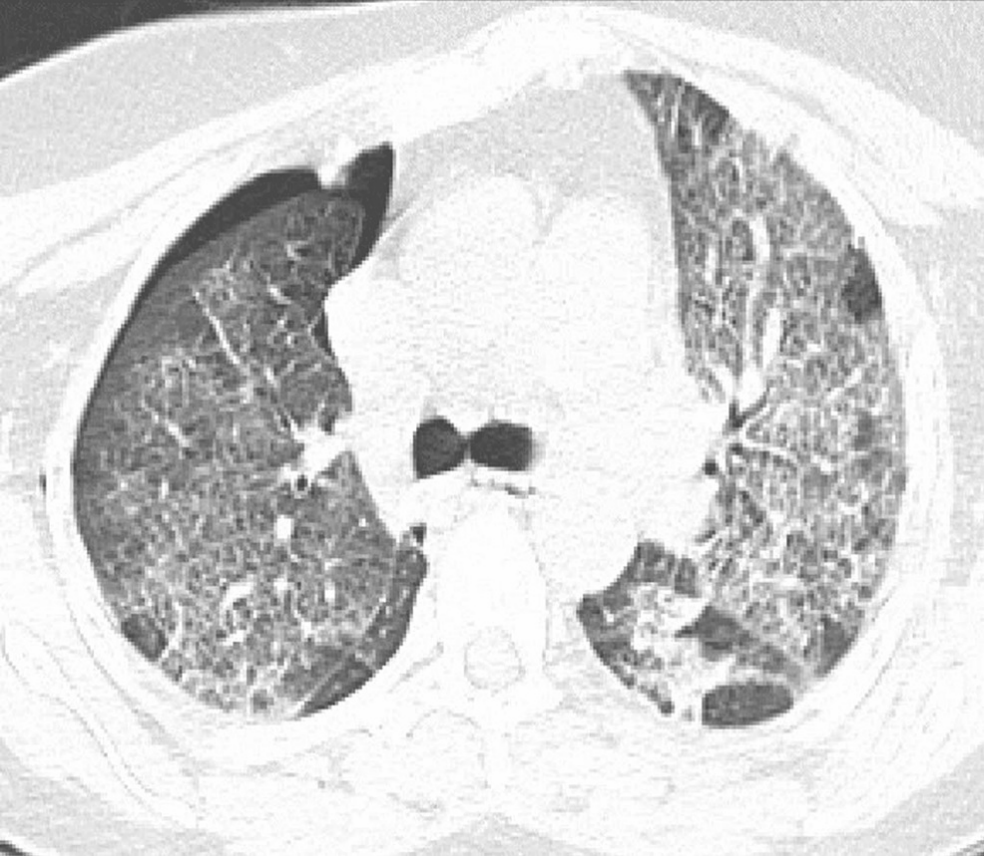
Chest CT showing crazy-paving pattern of bilateral diffuse ground glass infiltrates with smooth interlobular septal thickening and right-sided pneumothorax.
